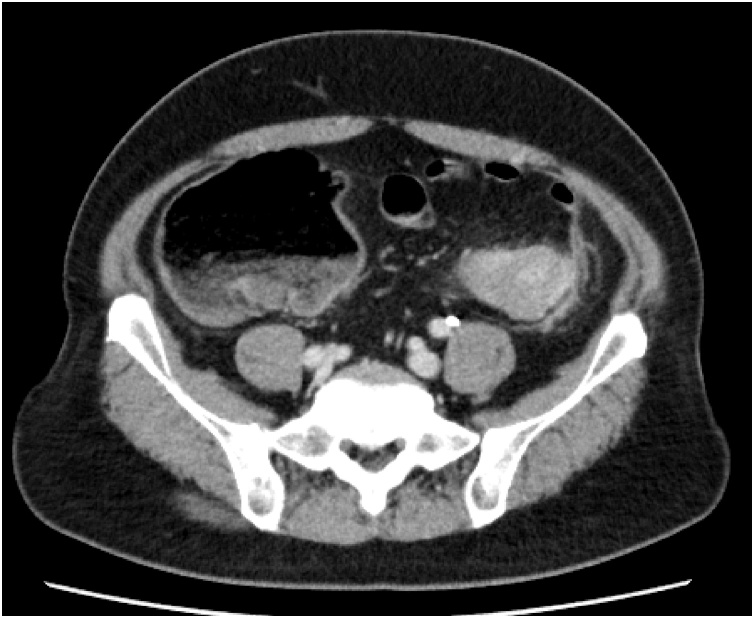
Contrast-enhanced abdominal computed tomography showed a marked thickening and circumferential stenosis in the descending colon.
